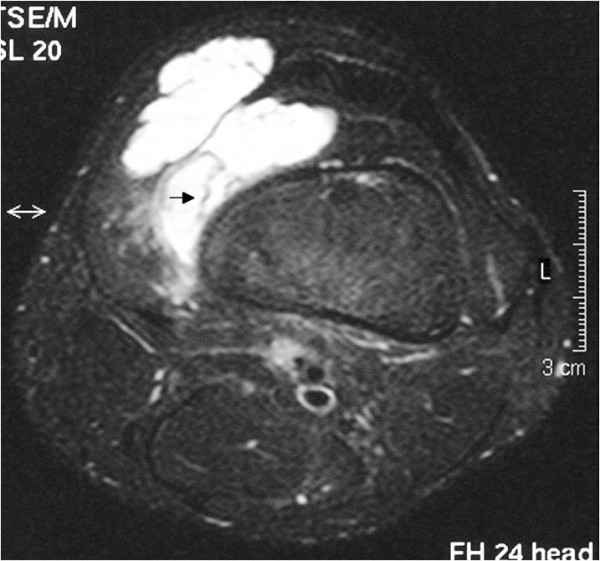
Axial T2-weighted image with fat suppression technique shows the tumour with a high signal intensity in the exact size and extent. A characteristic lace-like pattern (black arrow) and the tumour's extension into the vastus medialis muscle is seen.
